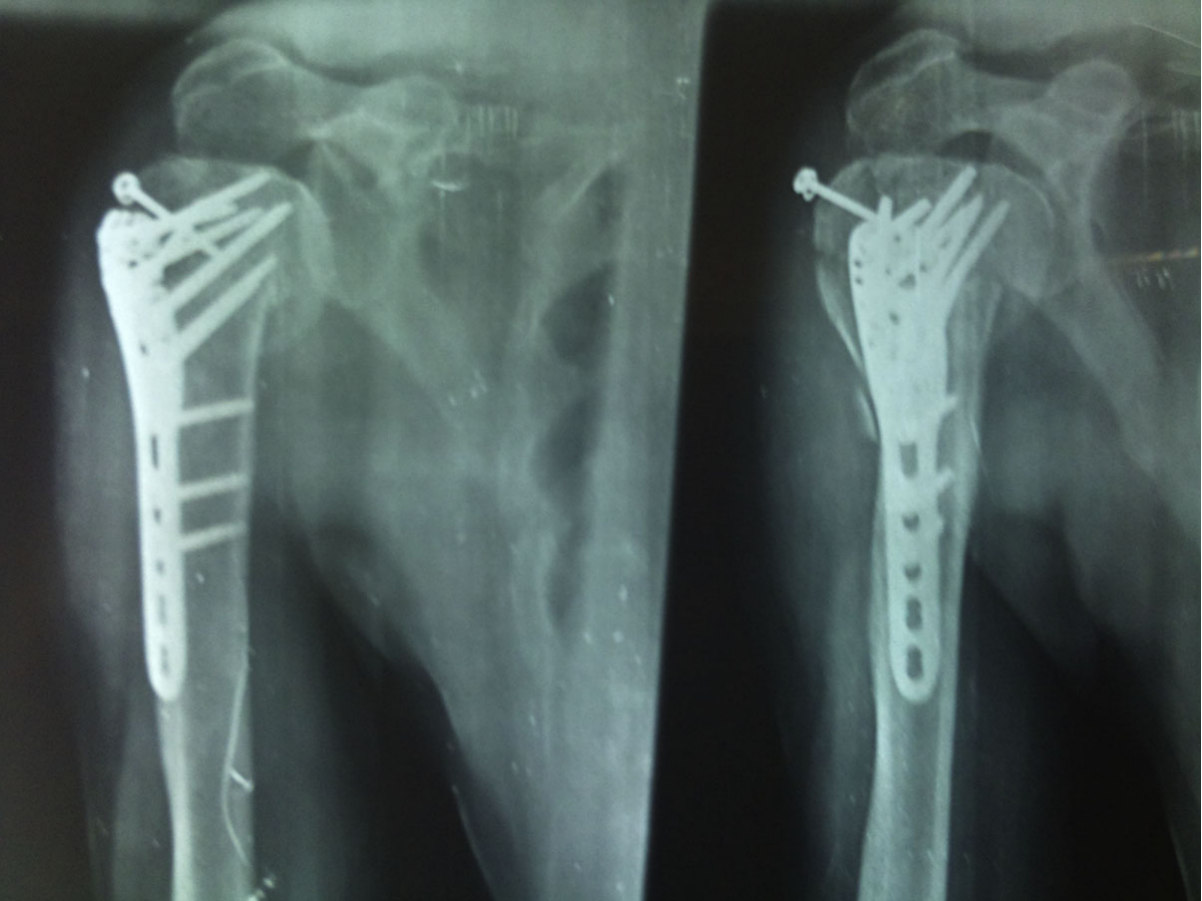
Varus collapse in a patient on follow up X-rays, both AP and lateral.
